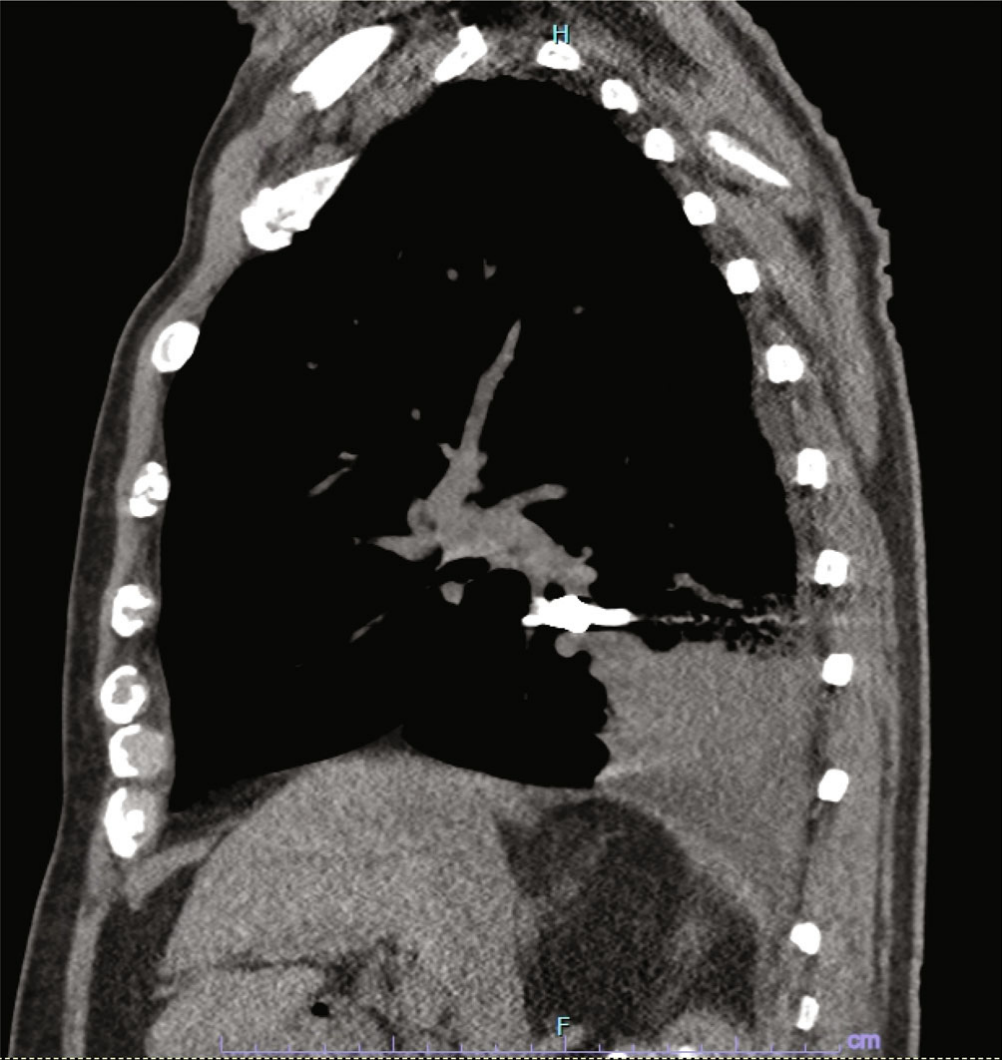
CT chest (sagittal view) with a metallic object and RLL consolidation.
